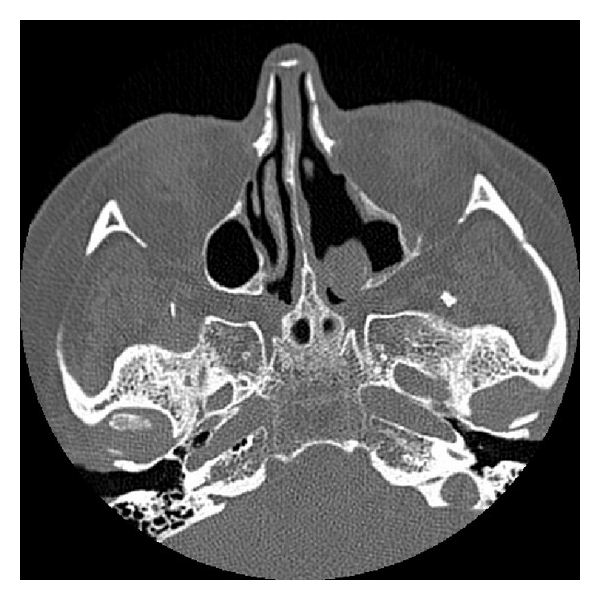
CT scan taken in 2007 showing evidence of surgery in the patient's left nasal cavity. The lesion has been removed, and the maxillary ostium is widened.
